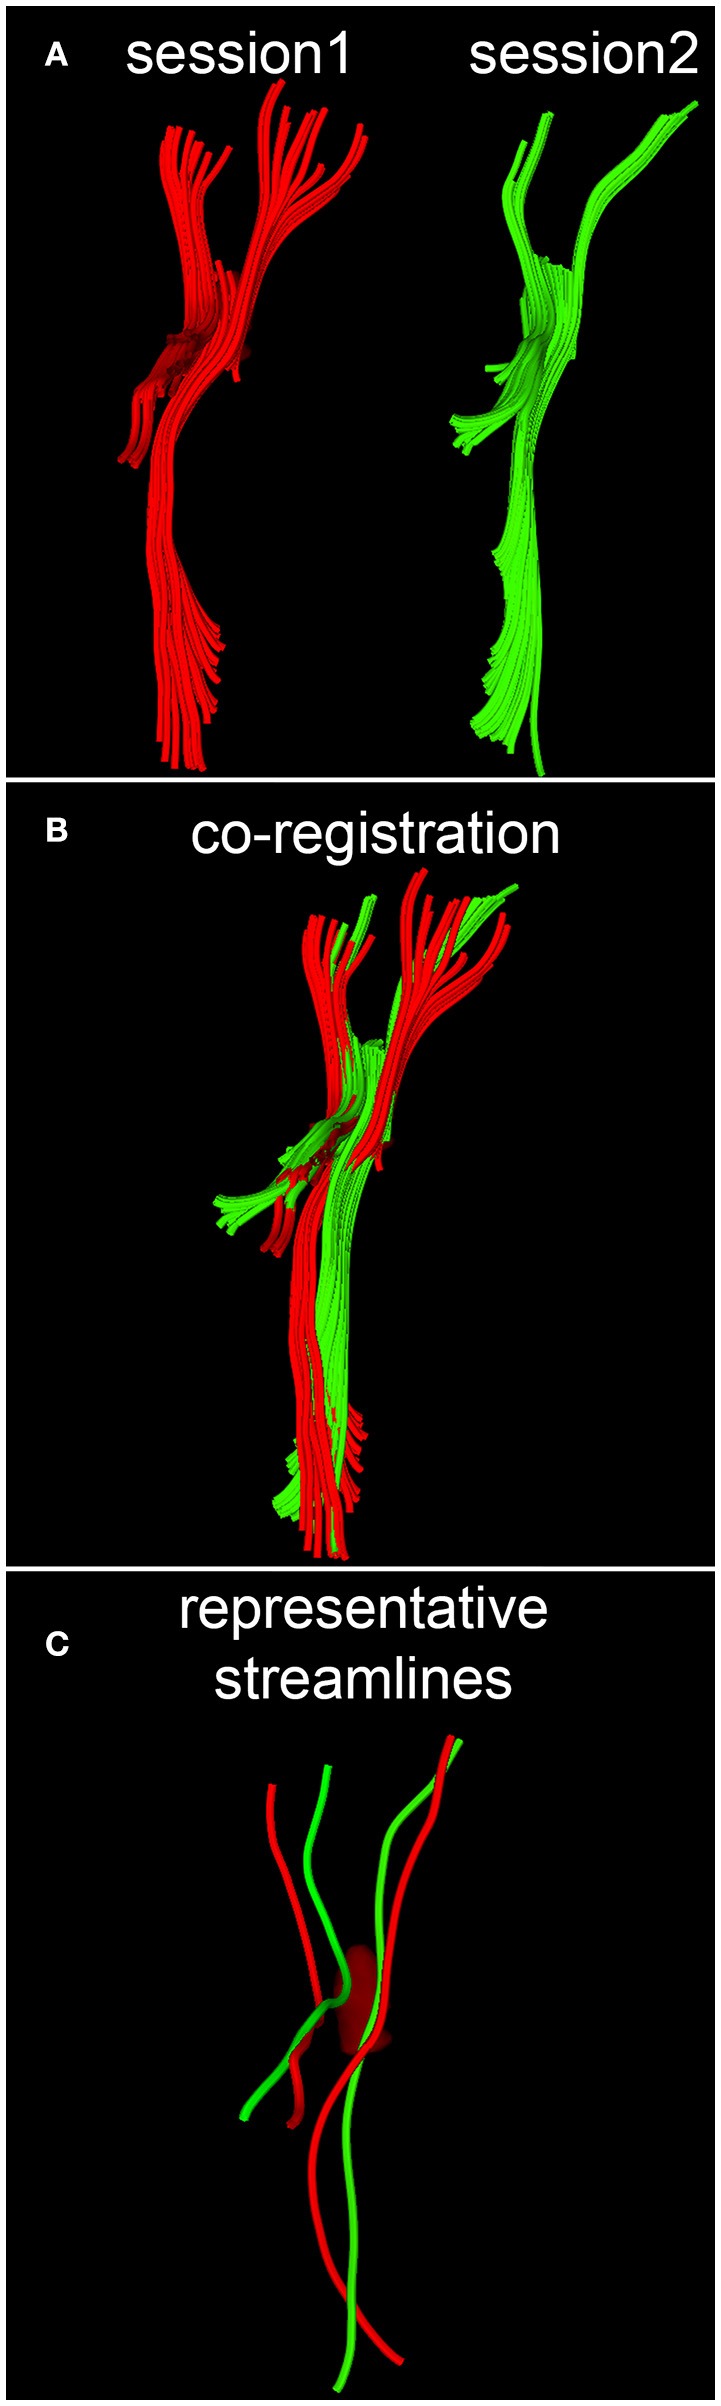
Conceptual representation of streamline matching, which involves (A) the reconstruction of DTI streamlines from DWI volumes acquired during each session, (B) the co-registration of streamlines within each subject, and (C) the selection of a streamline which is representative of a bundle's spatial trajectory.
